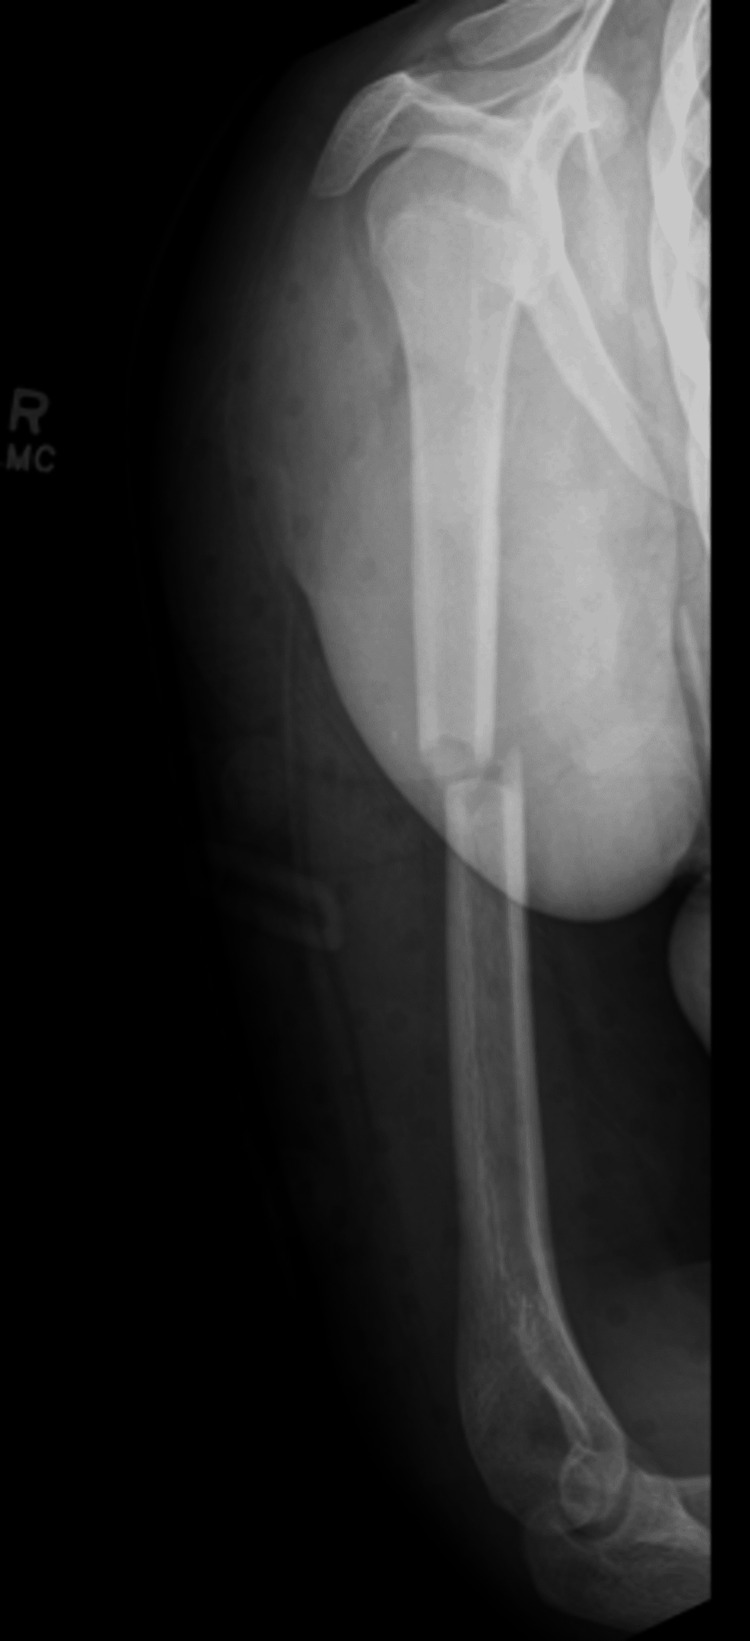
Lateral radiograph of the right humerus at six weeks post-injury. Redemonstration of transverse humeral shaft fracture. No callus formation noted. Fracture appears to maintain good alignment.
